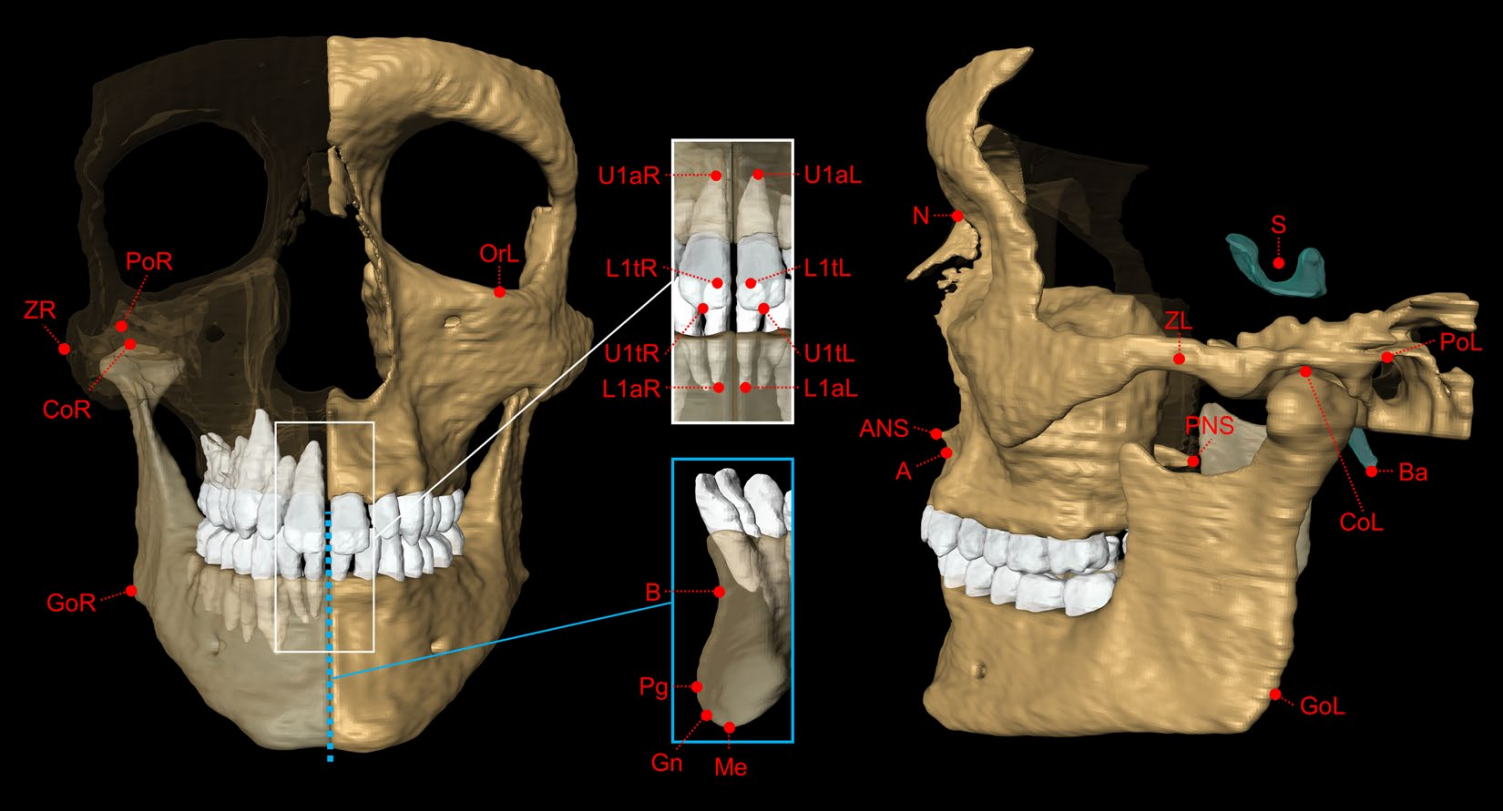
Cephalometric landmarks used in the present study. For each MRI dataset, 27 cephalometric landmarks were determined on multiplanar images. The 3D skull segmentation shown in this figure was generated from 3D MRI of volunteer #2 using Amira software (Version 6.4.0, Thermo Fisher Scientific, MA, USA). Definitions of cephalometric landmarks: N = Nasion (most anterior point of the frontonasal suture); S = Sella (geometric center of the pituitary fossa); Ba = Basion (lowest point on the anterior border of the foramen magnum); CoL/CoR = left/right Condylion (most superior point of Condyle); GoL/GoR = Left/right Gonion (midpoint on the curvature of the angle of the mandible); PoL/PoR = Left/right Porion (Most superior point of the external auditory meatus); OrL = Left Orbitale (most inferior point on the infraorbital margin); ZL/ZR = Left/right Zygion (Most lateral point of the zygomatic arch); ANS = Anterior nasal spine; PNS = Posterior nasal spine; A = Point A (point of maximum midline concavity on the maxilla); B = Point B (point of maximum midline concavity on the mandibular symphysis); Pg = Pogonion (most anterior point of mandibular symphysis); Me = Menton (most inferior point of mandibular symphysis); Gn = Gnathion (Midpoint between Pg and Me); U1tL/U1tR = Tip of the crown of the left/right first upper incisor; U1aL/U1aR = Apex of the left/right first upper incisor; L1tL/L1tR = Tip of the crown of the left/right first lower incisor; L1aL/L1aR = Apex of the left/right first lower incisor; Definitions of planes: N_ANS_PNS = Midsagittal plane defined by the landmarks N, ANS and PNS; PoL_PoR_OrL = Frankfort horizontal plane defined by the landmarks PoL, PoR and OrL.
